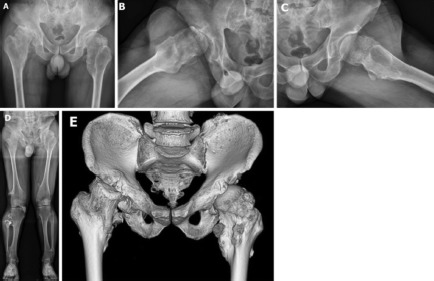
(a) Anteroposterior pelvic radiograph showing femoral head collapse and subluxation, with exostoses present on the inferomedial femoral neck on both sides. (b) Right femoral head lateral radiograph. (c) Left femoral head lateral radiograph. (d) Teleroentgenogram of the lower extremity showing several exostoses in the periarticular region. (e) 3D-scan confirmed the exact position of the proximal femoral exostosis.
